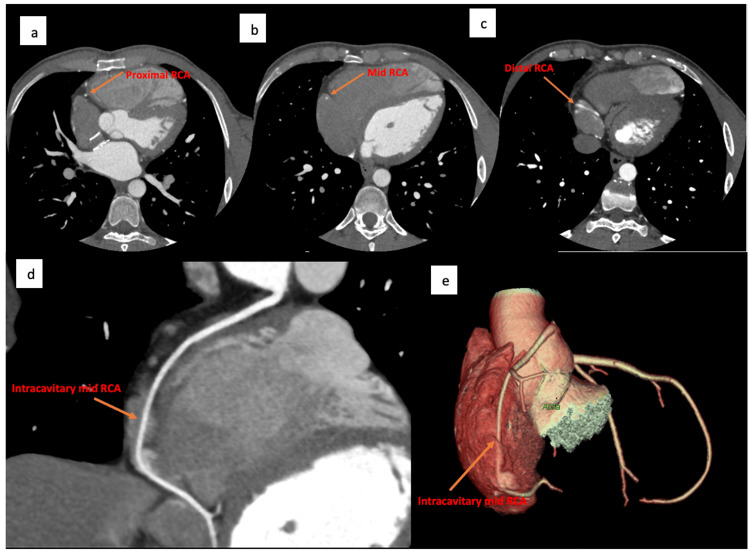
Axial, curved multiplanar reformatted as well as 3D volume rendering images from CT coronary angiogram demonstrate (a) Normal course of the proximal RCA segment along the right atrioventricular groove surrounded with epicardial fat, and (b, c, d, e) Intracavitary course of the mid and distal segments of the RCA within the right atrium. RCA: right coronary artery
